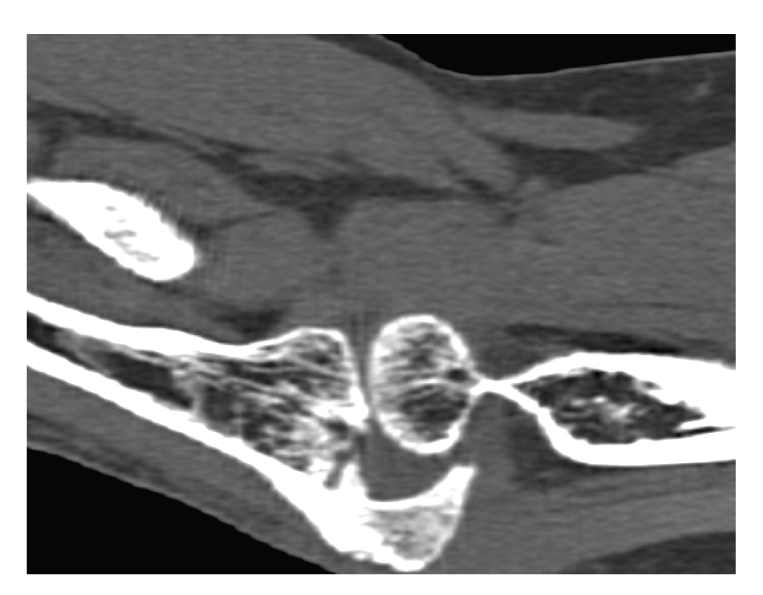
Computed tomographic evaluation revealed depression of the articular surface of the trochlear notch and shortening of the proximal ulna. She had an incongruent ulnohumeral joint leading to impingement and loss of function. The articular surfaces of the radio-capitellar joint remained opposed.
